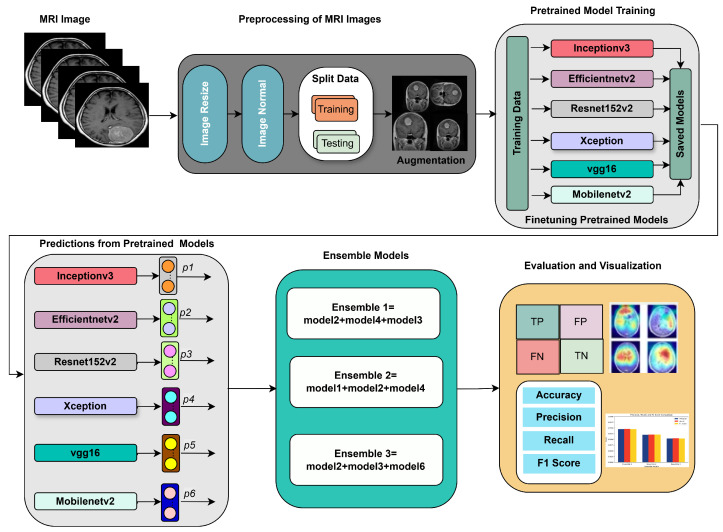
Overview of the proposed transfer learning-based ensemble framework, including MRI preprocessing, feature extraction using multiple pretrained models, fine-tuning, ensemble learning, and final classification.
